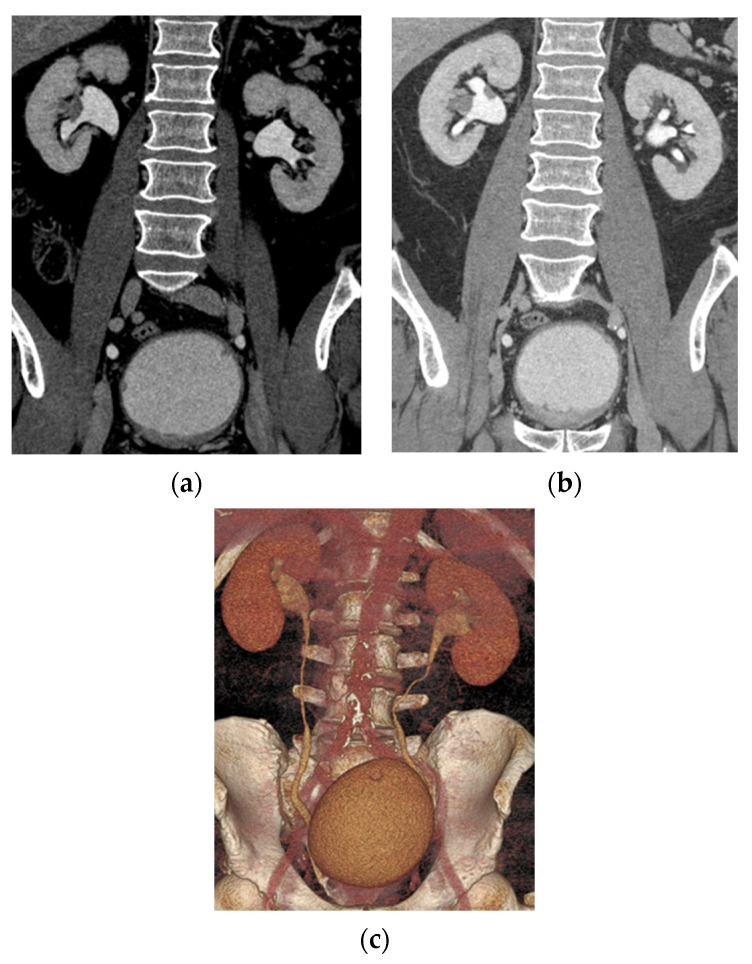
CT urogram showing synchronous upper and lower tract urothelial carcinoma. (a) Coronal CT image in the excretory phase shows multiple small bladder lesions (solid white arrow) and synchronous upper tract disease (dashed white arrow) (b) Coronal CT image slightly posteriorly, showing multiple upper tract lesions. (c) Coronal 3D volume rendering redemonstrates the findings with another bladder lesion (black arrow).
